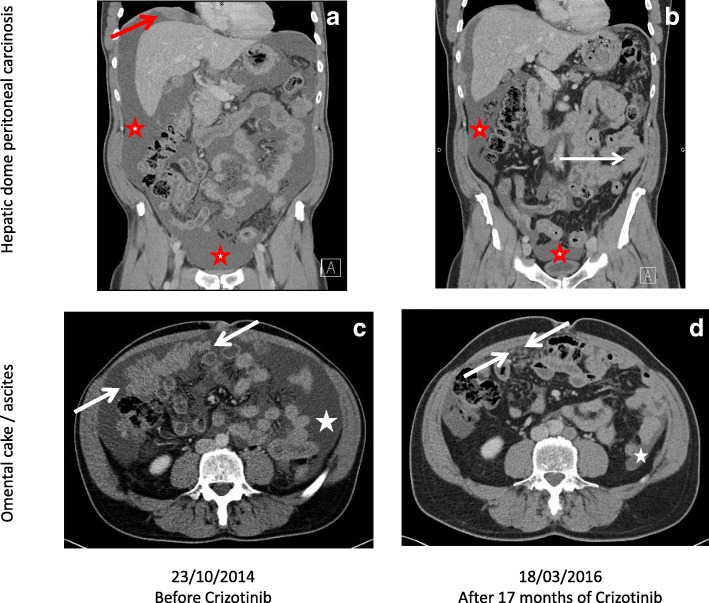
Radiological comparison of abdominopelvic CT scans before crizotininb (a, c) and after 17 months of crizotinib (b, d), in coronal (a, b) and transversal plane (c, d)
